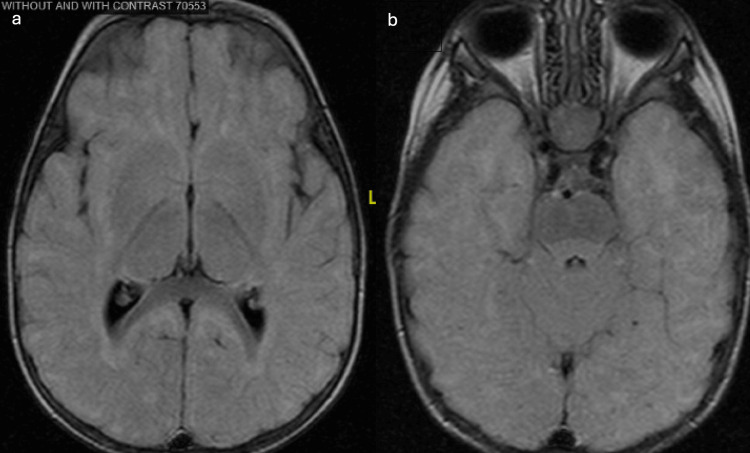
Brain magnetic resonance imaging in 2013 a. MRI results demonstrate a grossly normal examination of the brain. There is no evidence of restricted diffusion, abnormal susceptibility, or suspicious enhancement. No focus of abnormal parenchymal signal is demonstrated, indicating a normal degree of myelination for the patient’s age. b. Incidentally noted is mucosal thickening of the right greater than left maxillary sinuses. There is mild hypertrophy of the adenoids and enlargement of the palatine tonsils.
